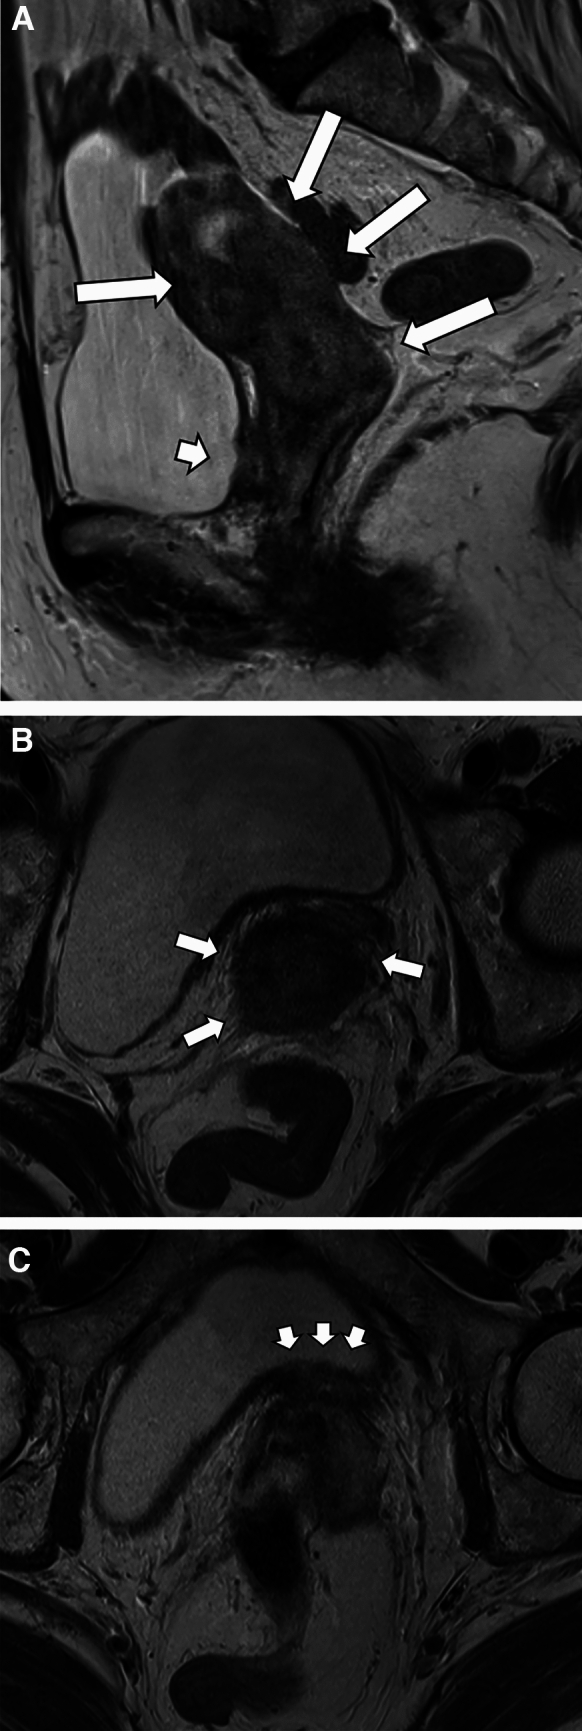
A representative case of unexpectedly advanced gastric-type endocervical adenocarcinoma in her 70s preoperatively diagnosed with FIGO IIB. MRI shows a cervical tumor extending to the lower uterine body (A, T2-weighted sagittal image, long arrows) and invading the anterior (A, a short arrow) and bilateral parametrium (B, T2-weighted oblique axial image: arrows). The tumor disrupts the bladder wall but does not extend into the lumen (C, T2-weighted oblique axial image, short arrows). In this case, no bladder mucosal invasion was diagnosed preoperatively, and cystoscopy also showed no evidence of mucosal invasion. Anterior pelvic exenteration was performed. It was pathologically staged as T4aN1: invasion to bilateral parametrium, mucosa of bladder and left ureter, and bilateral pelvic lymph node metastases
